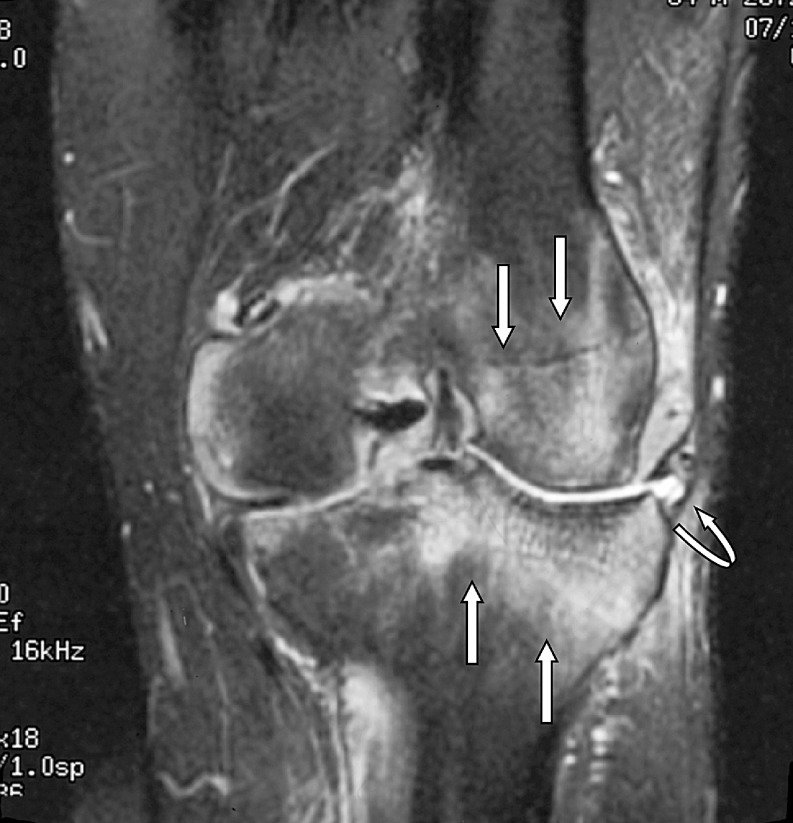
Global edema with a total knee joint replacement in a 65-year-old male patient. The radiograph demonstrated absence of joint space in the medial and lateral compartments, and irregularity of the subchondral bone plate of the lateral femoral condyle. Coronal fast fat-suppressed T2-weighted image (TR 3000/TE 72/256 × 192) through the knee shows extensive edema in the lateral femoral and tibial condyles, extending into the metaphyses (*straight arrows*). Note also the small extruded body of the degenerative and torn lateral meniscus (*curved arrow*)
